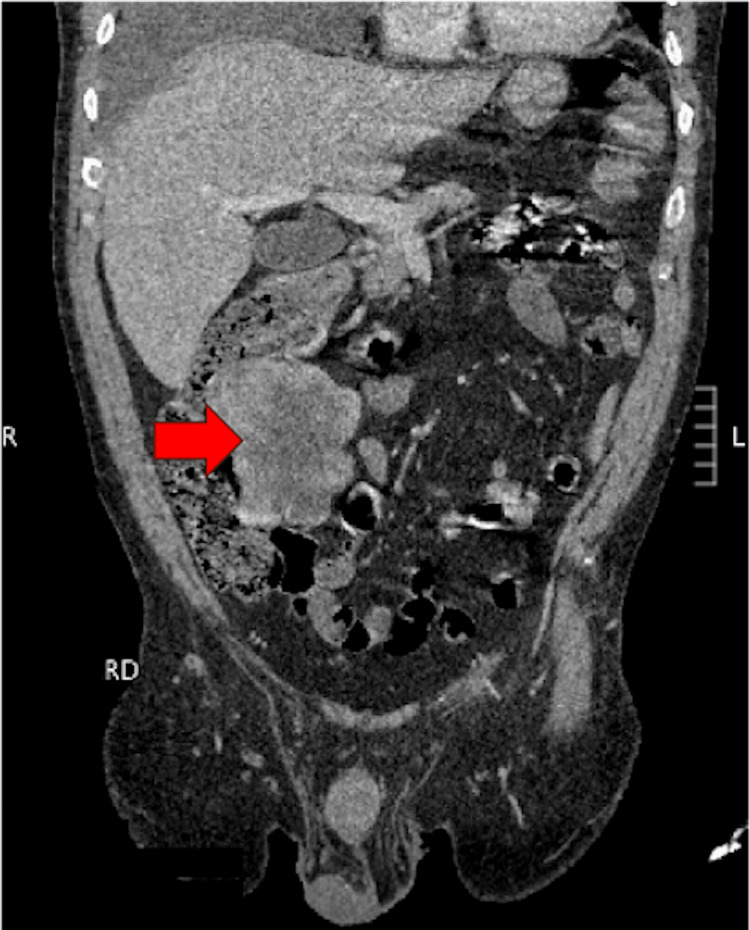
Coronal view of contrast-enhanced computed tomography (CT) of the abdomen demonstrating a well-circumscribed mesenteric mass adjacent to the ascending colon (red arrow). The image highlights the size and location of the lesion, consistent with metastatic involvement from an inflammatory myofibroblastic tumor.
